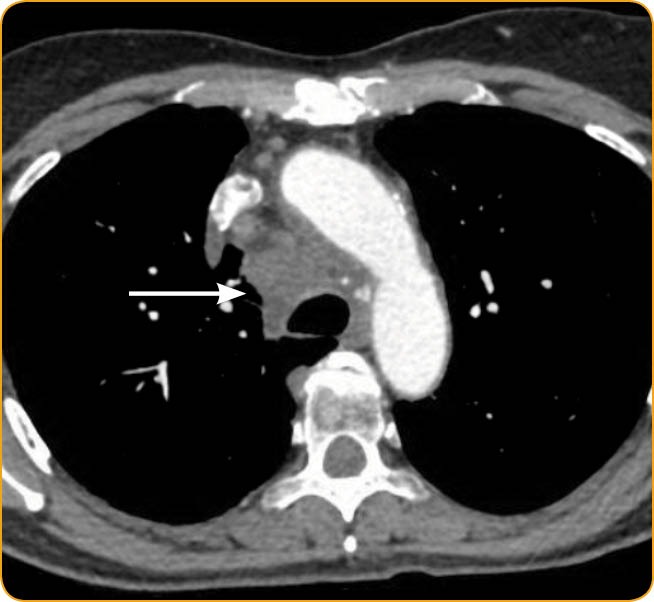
CT scan shows a large, 2.5-cm malignant mediastinal lymph node (arrow).
